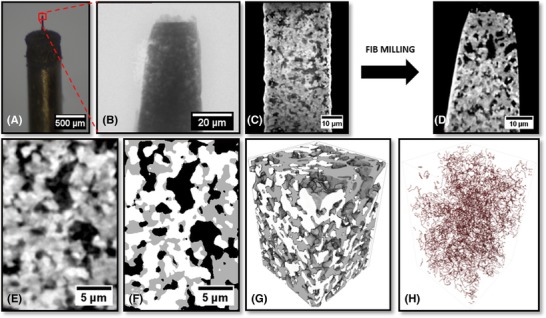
(A) Optical image of the new laser‐prepared pillar where the red square highlights the region of interest at the top of the pillar. (B) Corresponding radiograph of the top of the pillar. (C) Reconstructed vertical slice directly after laser micromachining. (D) Reconstructed vertical slice after further FIB milling. (E) Raw slice from usable volume after FIB milling. (F) Corresponding segmented image showing the three phases. (G) 3D reconstruction of full volume (white = YSZ, grey = nickel, transparent = pore volume), (H) 3D TPB map.
